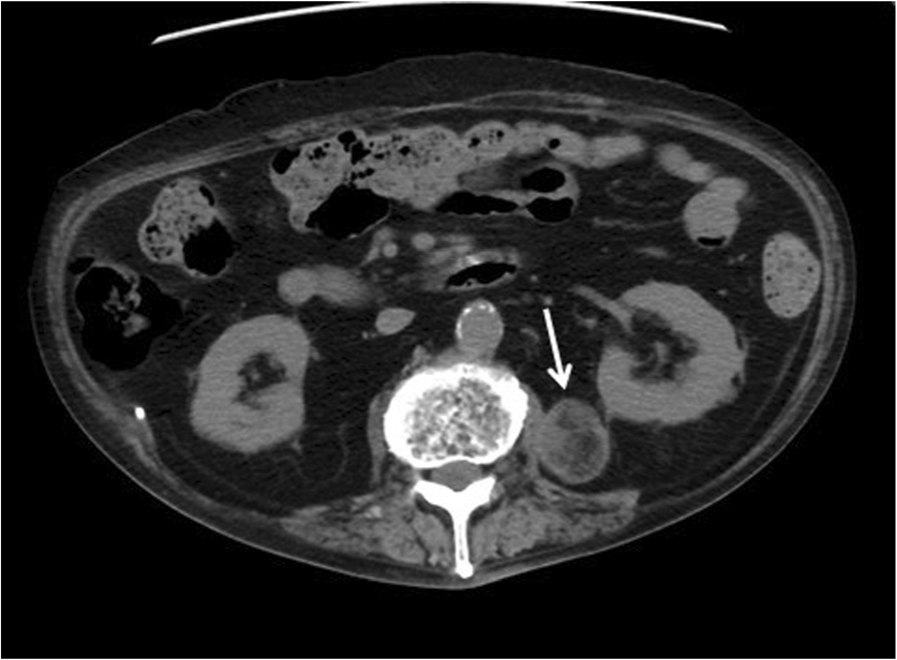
Abdominal CT image showing a 30 mm × 35 mm mass in the left retroperitoneal cavity
